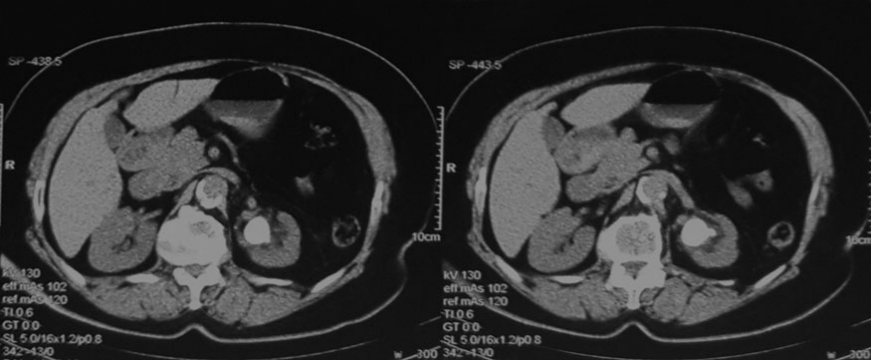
CT cross section slides of a 75-year-old female patient, showing large UPJ stone, thinning of left renal cortex, and renal pelvis dilation.
